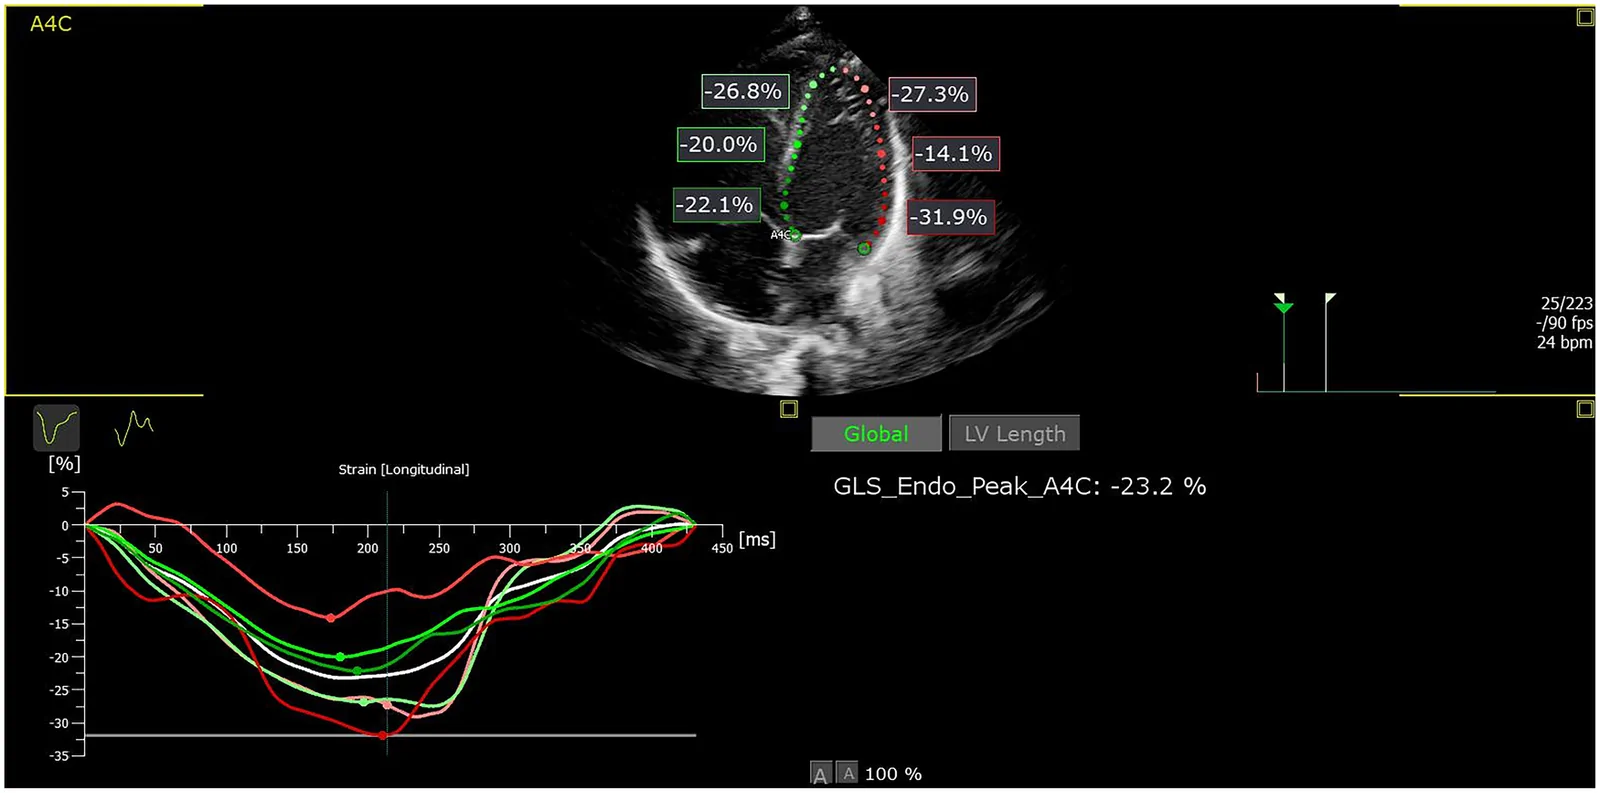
Neonatal autostrain left ventricle evaluation. GLS_Endo_Peak_A4C: left ventricle peak longitudinal strain from apical 4 chamber view.
